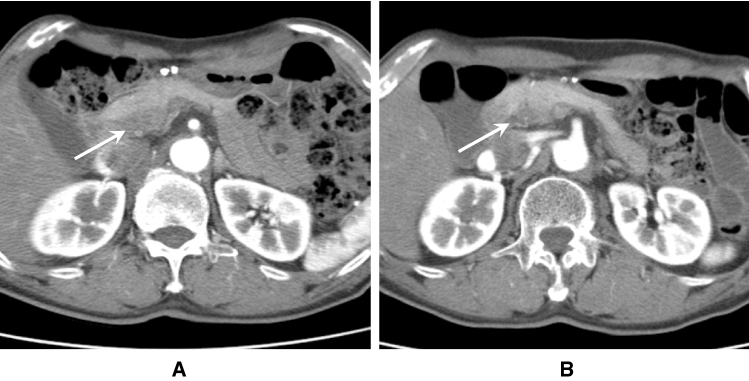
Contrast-enhanced CT images of focal AIP before and after corticosteroid therapy. A DCE-CT (pancreatic phase) shows a hypovascular lesion in the pancreatic head (arrow). B DCE-CT (pancreatic phase) after corticosteroid therapy reveals improvement of the pancreatic head lesion (arrow)
